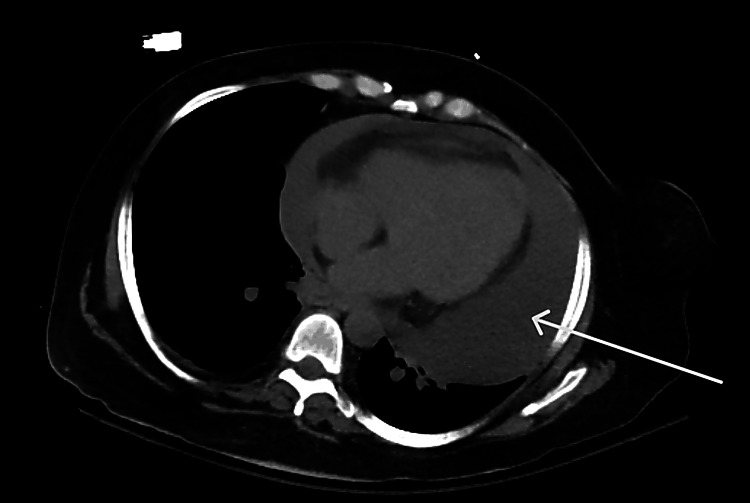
Axial CT scan (lower thoracic cut) showing a circumferential low attenuation surrounding the heart (arrow), compatible with a large pericardial effusion.
